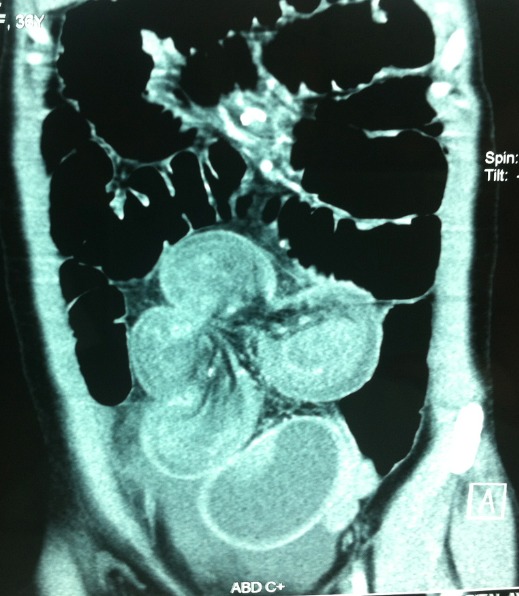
Abdominal CT- Scan, frontal slice, showing the intussusception and the volvulus of the small bowel
